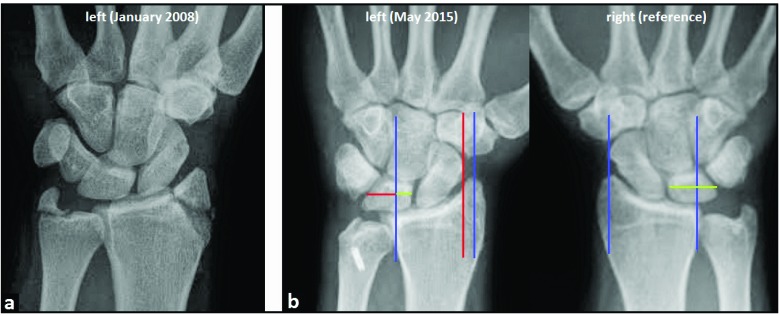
(Case Presentation, preoperative radiographic course over 7 years in comparison to the uninjured right wrist as reference: blue longitudinal and green transverse lines): (a) PA radiograph of the left wrist showing primary fractures of the radial and ulnar styloid without SLL disruption and without PUCT; (b) PA radiograph of the left wrist 7 years after primary injury showing PUCT type I involving the entire carpus (red longitudinal and transverse lines) in comparison to the uninjured right wrist, less than 50% of the left lunate bone articulates in its fossa of distal radius whereas more than 50% of the right lunate bone articulates with the opposing bone, there is no positive ring sign in left scaphoid bone detecting scapholunate instability, note that the 2 screws for ORIF of radial and ulnar styloid were formerly removed.
